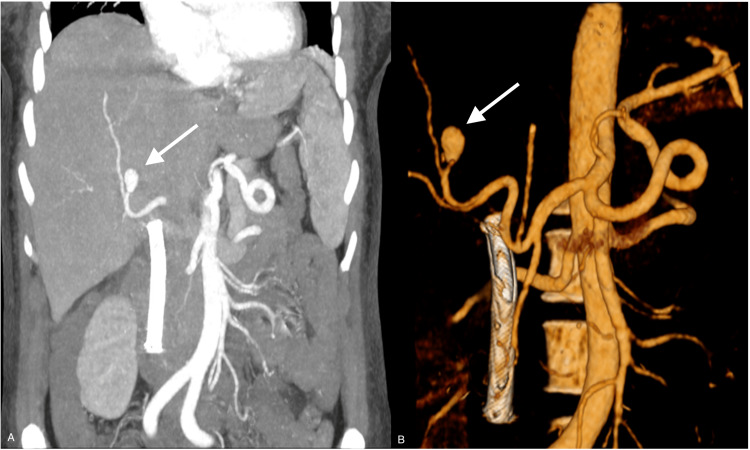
CTA (A) and 3D angiographic study (B) reconstruction of the abdomen showing the presence of a pseudoaneurysm is documented in a segmental branch of the right hepatic artery with a diameter greater than approximately 12 mm (arrows); a biliary stent previously placed in the common bile duct can also be seen CTA: computed tomography angiography; 3D: three-dimensional
